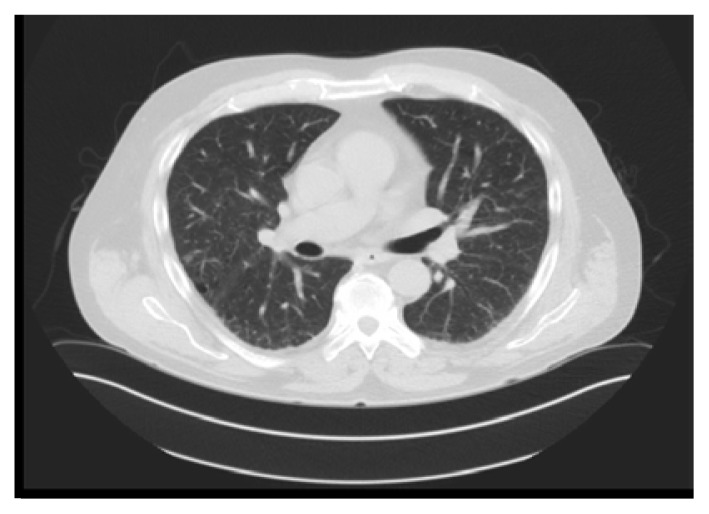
Comparative CT of the chest showed resolution of the right pulmonary nodules.
